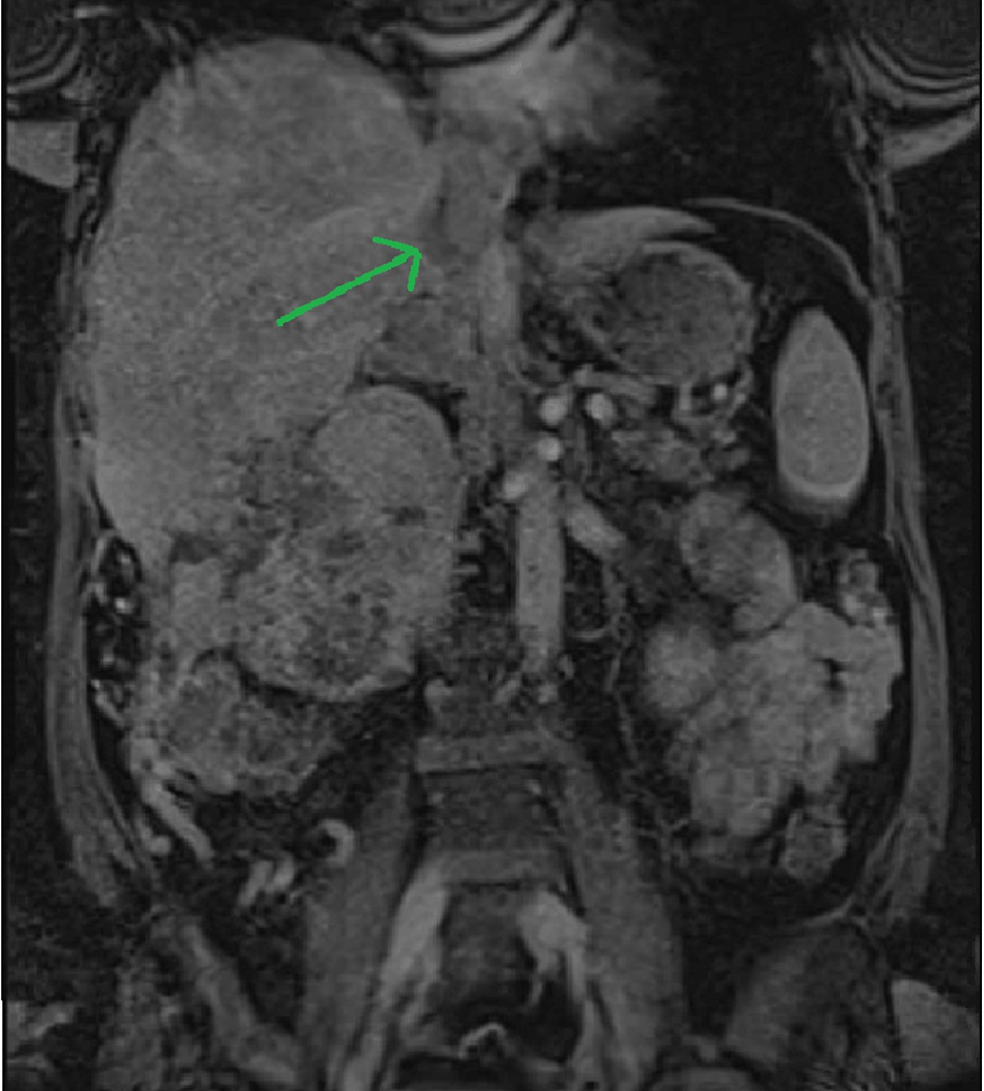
MRI showing vascular anatomy with TT in the IVC. TT: tumour thrombus, IVC: inferior vena cava, MRI: magnetic resonance imaging.
